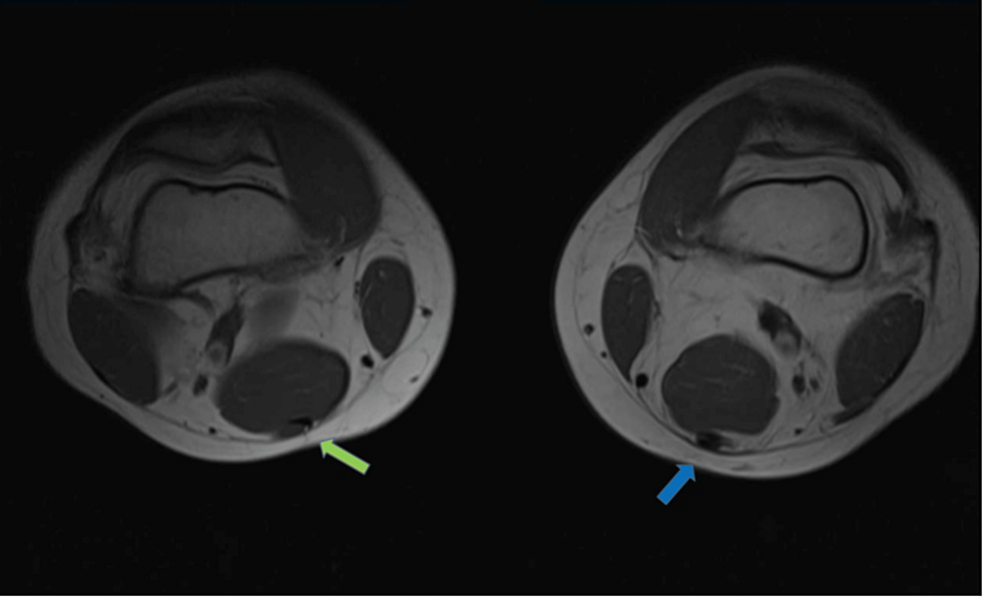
Axial T1 magnetic resonance imaging: Distal third left thigh level. Thickening of the distal third of the left semitendinosus tendon (blue arrow) in comparison to the normal appearance of the right semitendinosus tendon (green arrow).
